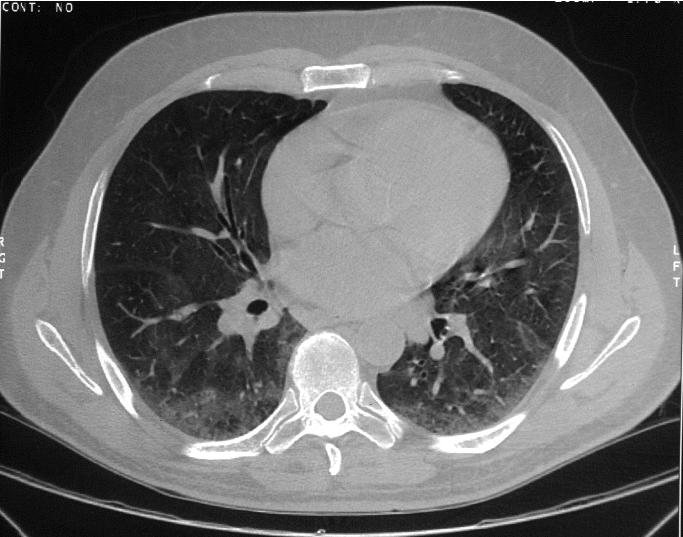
Thin-section CT scan of the chest showing ground glass opacities in the lung parenchyma, indicating interstitial inflammation and/or fibrosis.
